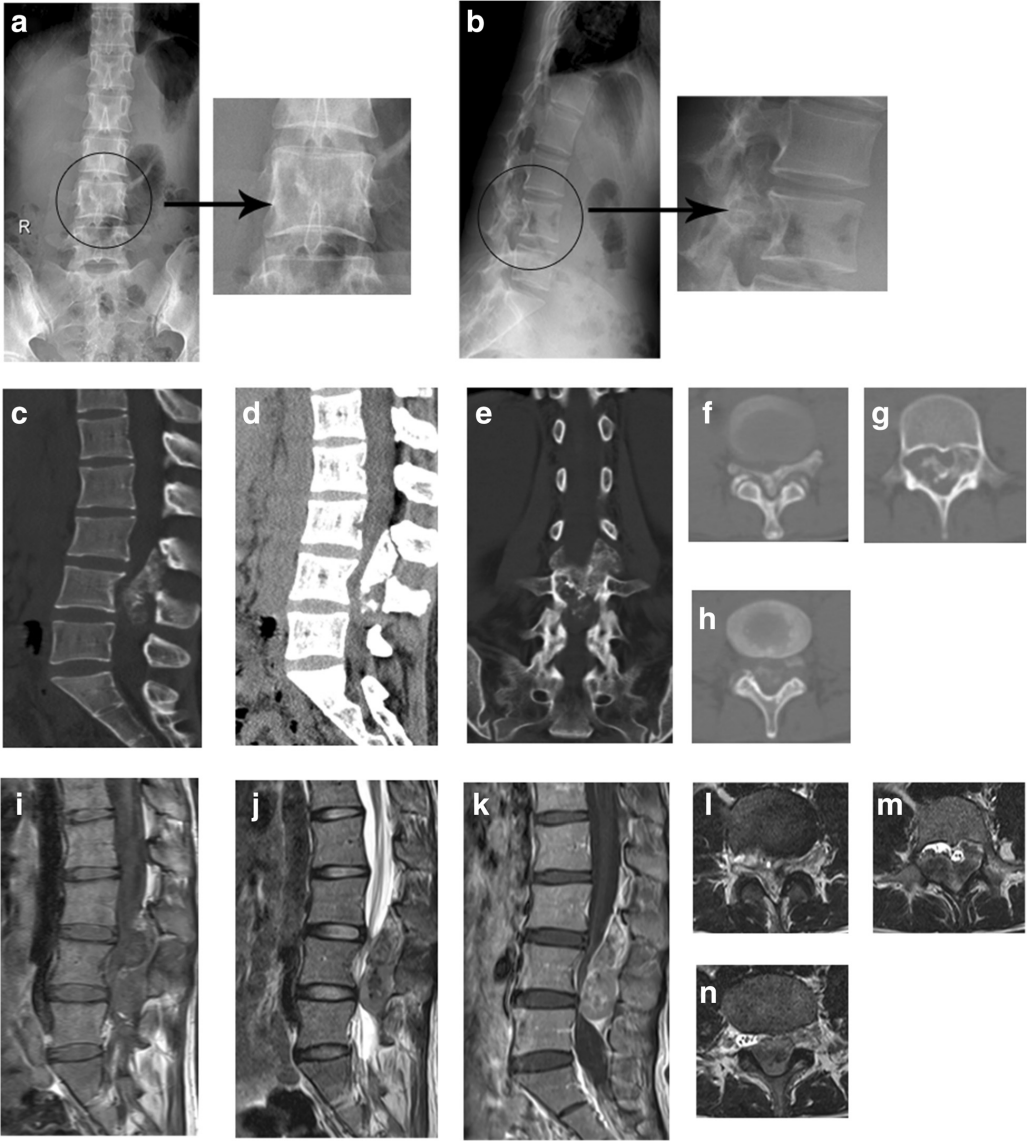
Images of X-rays and CT and MRI scans before operation. a, b Images of preoperative X-rays show unclear edge of left vertebral pedicle and posterior vertebra body of L4. c–h Preoperative CT images find massive calcifications within the mass. Images of f–h represent the level of L3, L4, and L5, respectively. i–k Images of preoperative MRI. Sagittal T1WI (i), T2WI (j), Gd-enhanced T1WI (k), and axial T2-WI (l–n) reveal a dumbbell tumor at the L3-5 level
